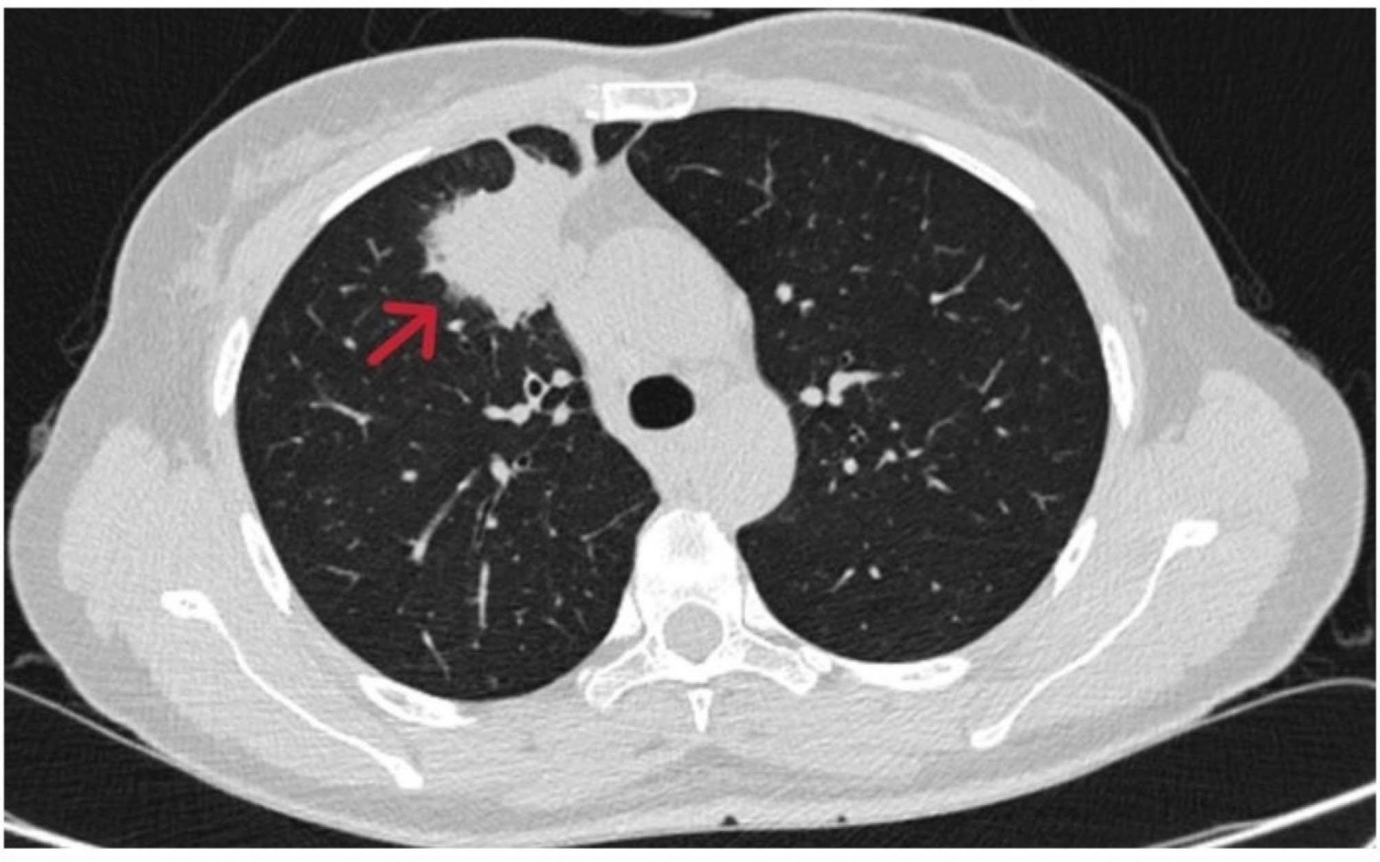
CT showed a 36.7mm *34.1mm mass with irregular shape, lobalation, spiculation, pleural indentation, and vessel convergence
